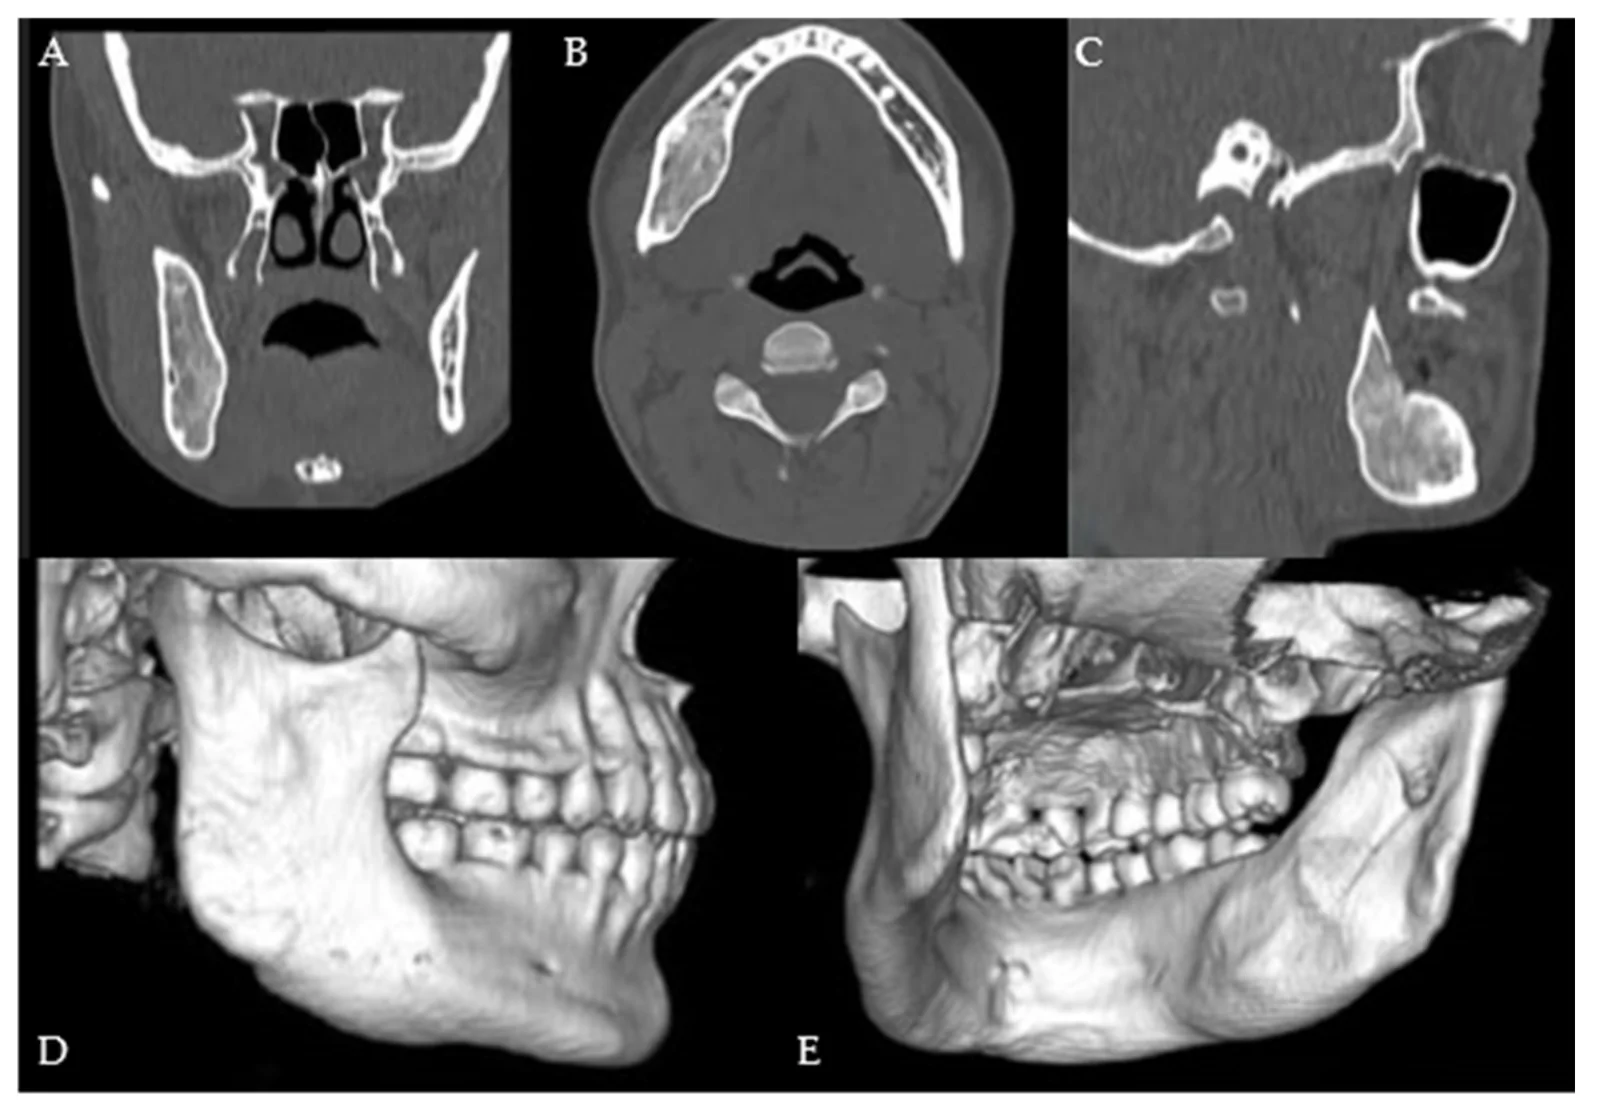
Cone-beam computed tomography (CBCT) at 2 years of follow-up. (A) Coronal, (B) axial, and (C) sagittal sections; (D) right lateral and (E) left posterior oblique views of the mandibular tomographic reconstruction, showing complete bone regeneration in the treated mandibular region without evidence of recurrence.
